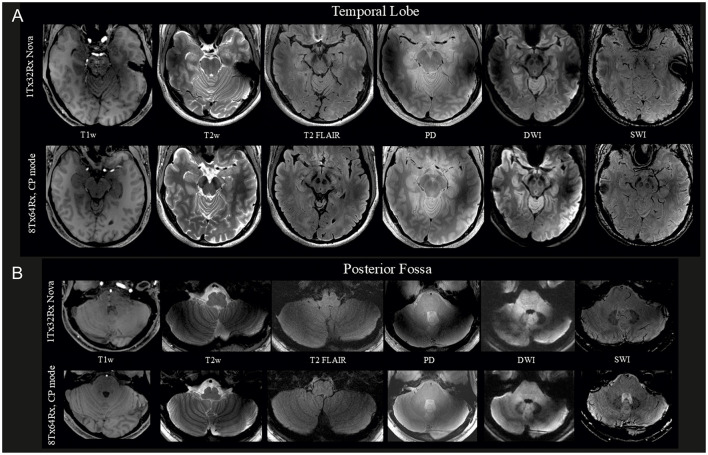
Comparison of images acquired in a healthy volunteer with various sequences (axial 3D T_1_-weighted, T_2_ TSE, T_2_ FLAIR, PD, DWI, and SWI) at the level of the temporal lobes (A) and cerebellum/posterior fossa (B), obtained respectively with the 1Tx32Rx coil (top rows) and 8Tx64Rx (CP) (bottom rows).
